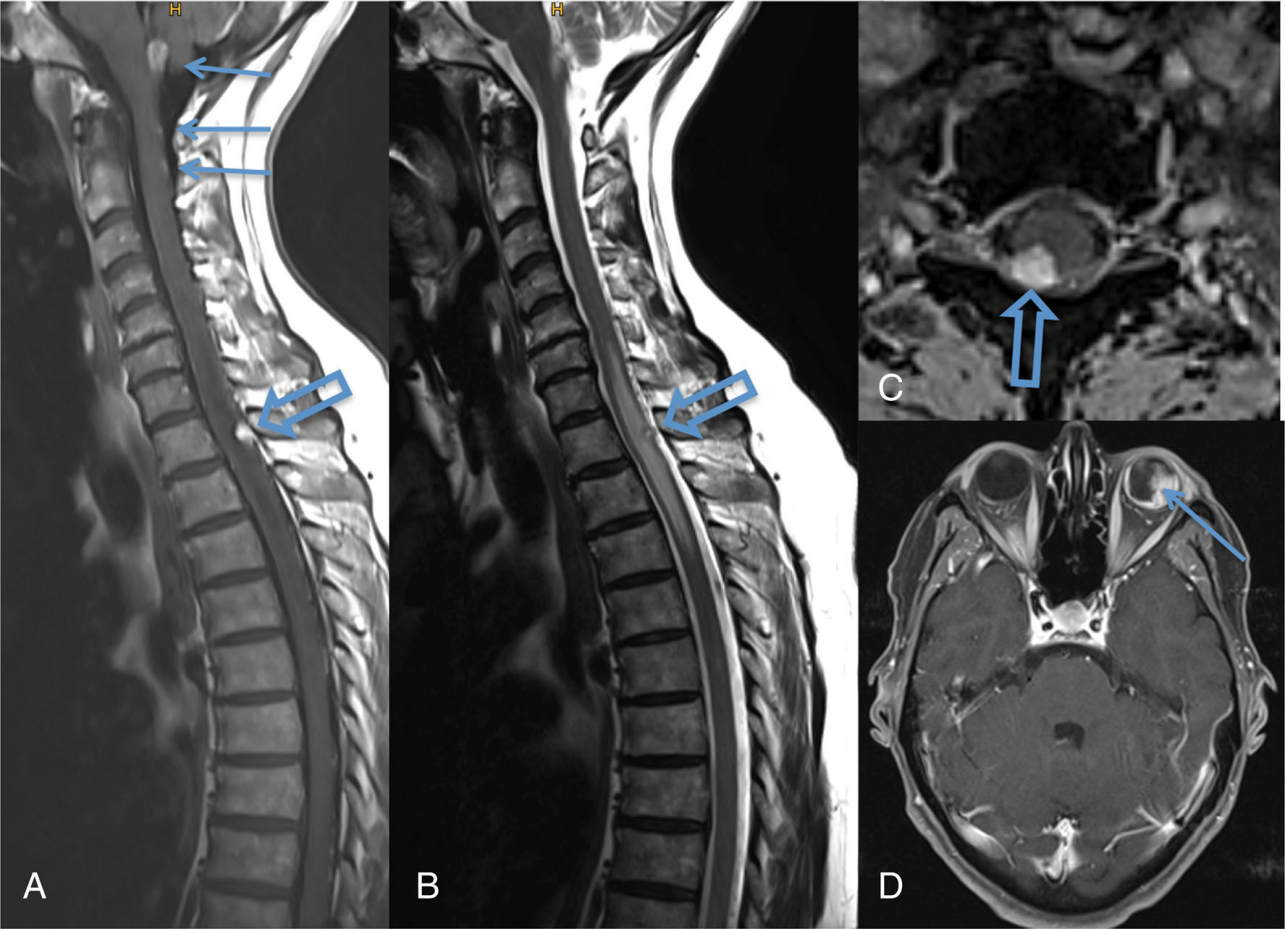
Patient with VHL disease and multiple hemangioblastomas in the obex and the dorsal aspect of the spinal cord. All lesions have a pial contact. The one at level Th1 (a–c: hollow arrow) has caused a spinal cord edema. Axial T1-weighted contrast-enhanced images with fat sat also show a left-sided retinal “angioma” (d: arrow)
